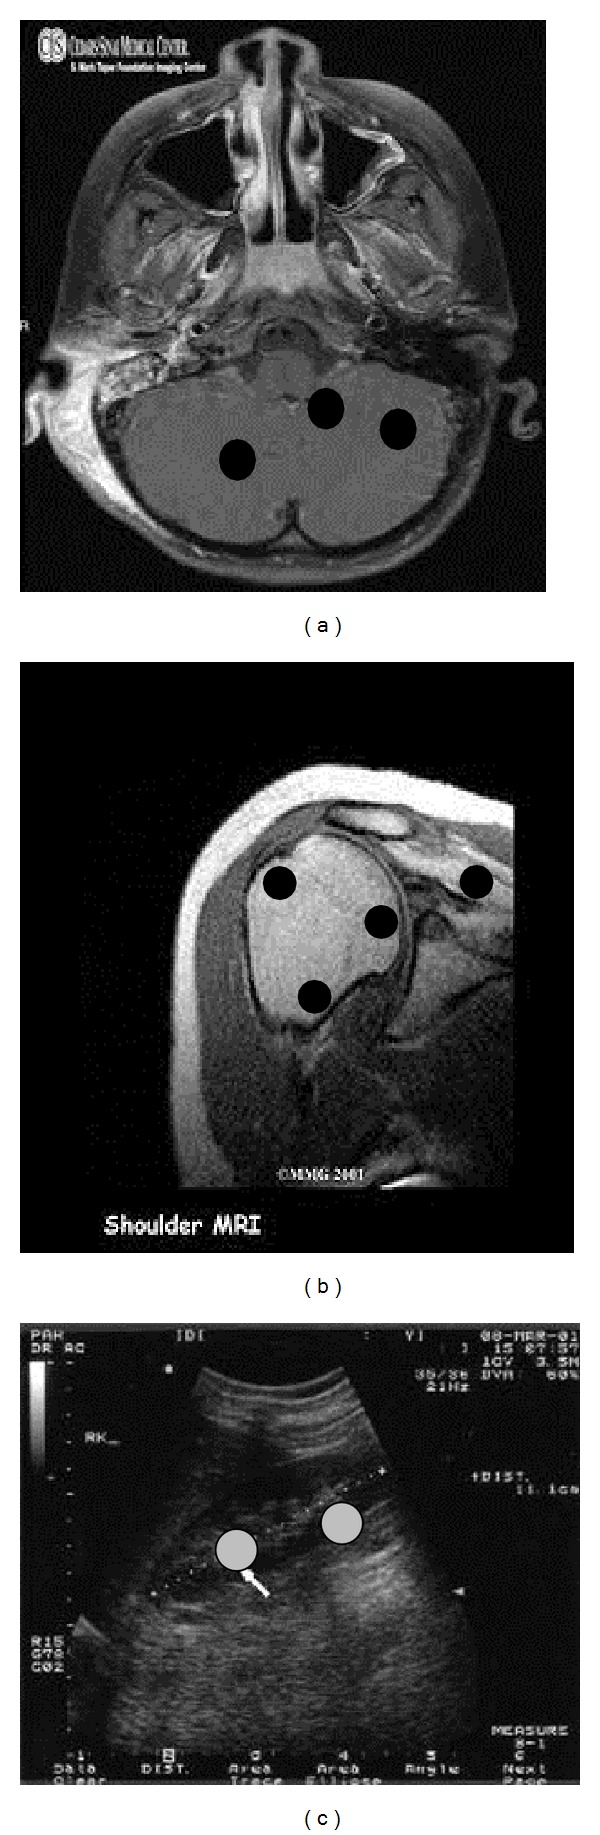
Watermarked medical images (from left to right: CT scan, MRI scan, and ultrasound) with tampers inside ROI.
